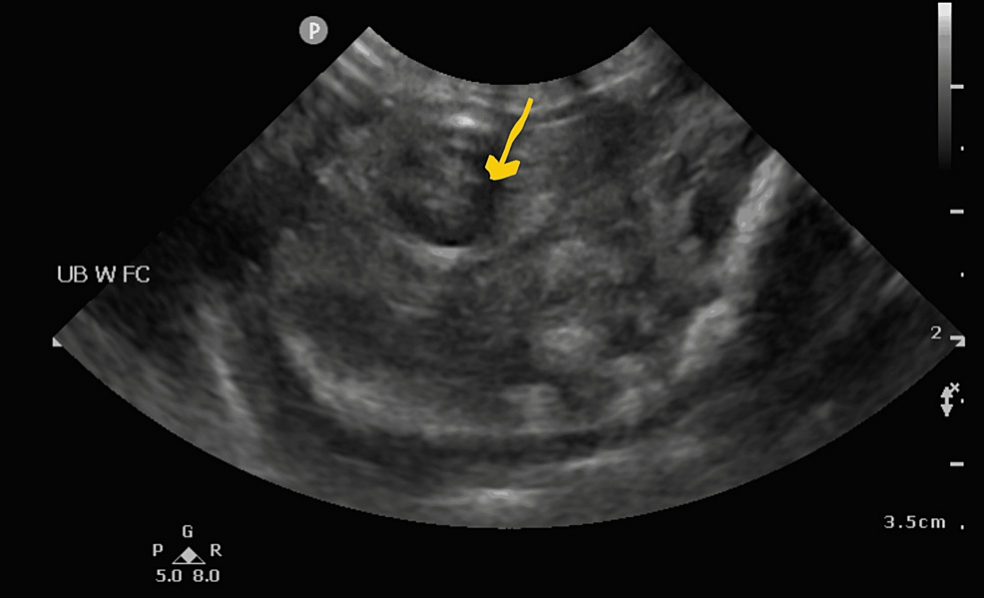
Ultrasound showing resolution of ascites post drainage
